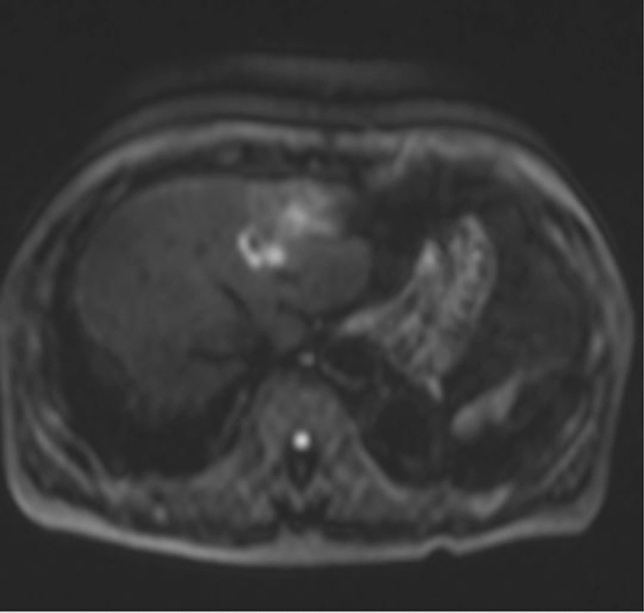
Two months later, MRI showed the size of the mass in S3 reduced on DWI
